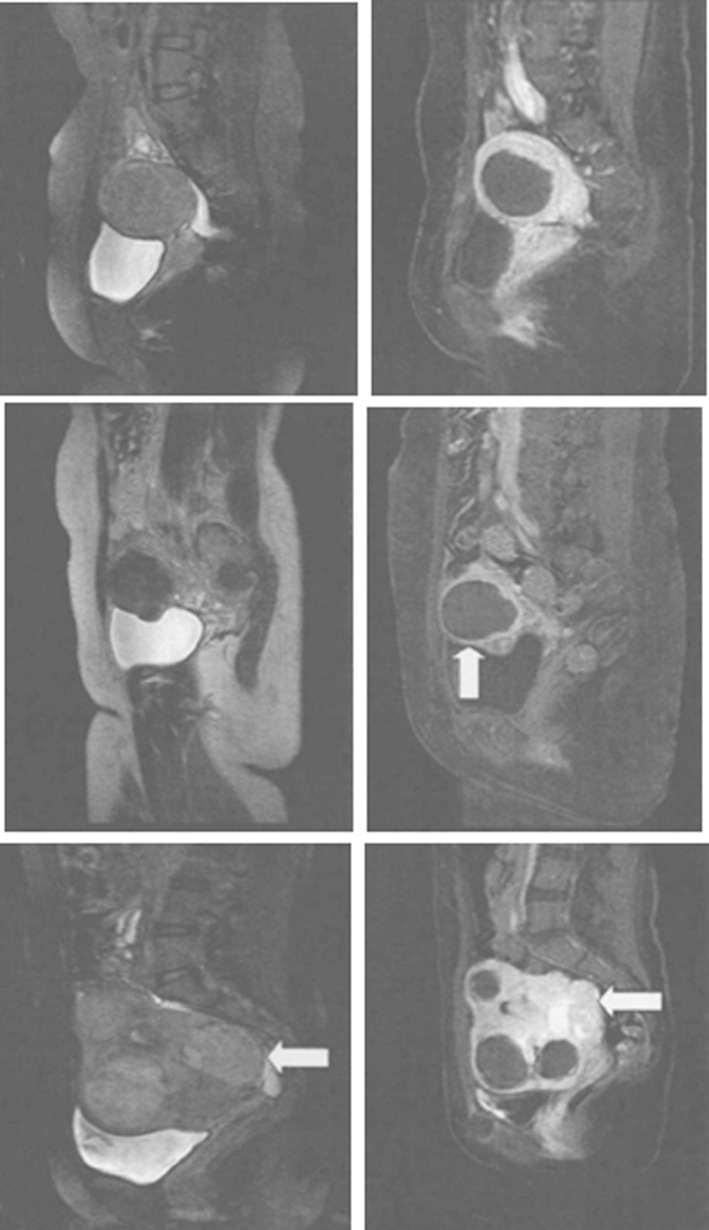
MRI images for different types of UFs. Left: Before HIFUA; right: after HIFUA. First row: intramural fibroid; second row: subserosal fibroid; third row:submucosal fibroid.
